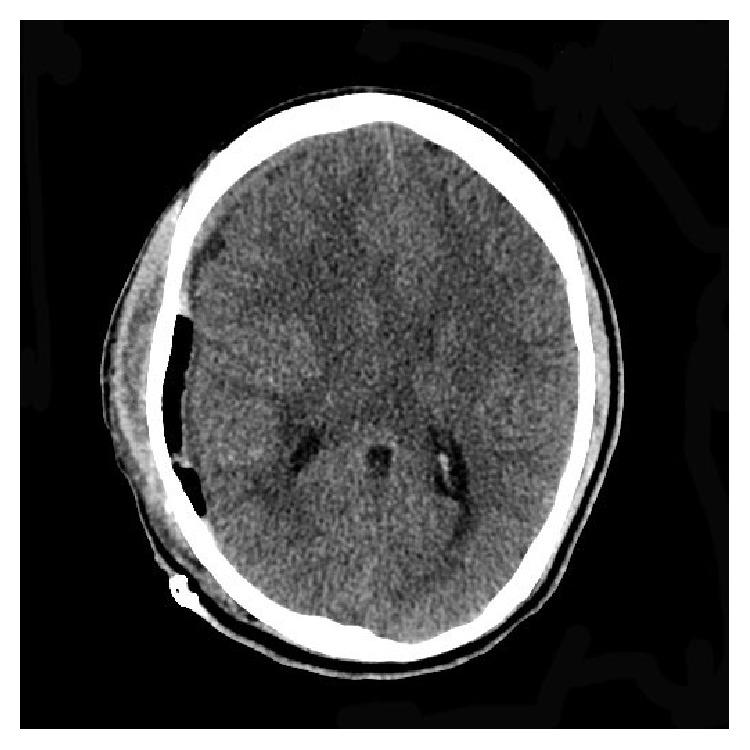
Postop CT showing resolution of epidural hematoma and postop changes on right.
